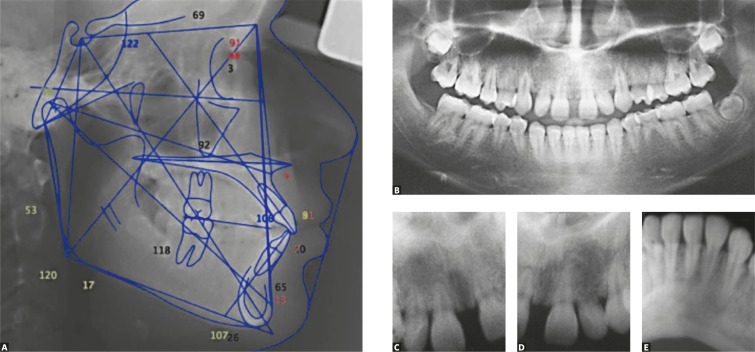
Initial radiographs. A) Cephalometric tracing; B) Panoramic radiograph; C)
